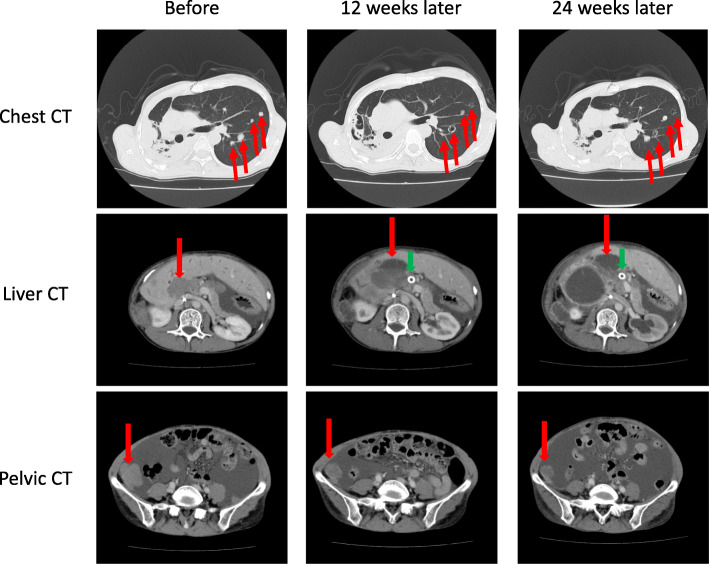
Computed tomography (CT) images showing the effect of pazopanib (800 mg/day). The upper, middle, and lower lines indicate chest CT, liver CT, and pelvic CT, respectively. The left, central, and right rows indicate before administration of pazopanib, 12 weeks later, and 24 weeks later, respectively. Red arrows and green arrows indicate tumors, and common bile duct stents, respectively. Chest CT images show that lung metastases reduced after 12 weeks of treatment and recurred after 24 weeks, in comparison with the first scan. Liver CT images show that liver metastatic tumors reduced and liquefied, while continuing the administration of pazopanib. Pelvic CT images show that the right pelvic dissemination tumor reduced, and there was a decrease in ascites after 12 weeks of treatment and an increase in ascites 24 weeks later
